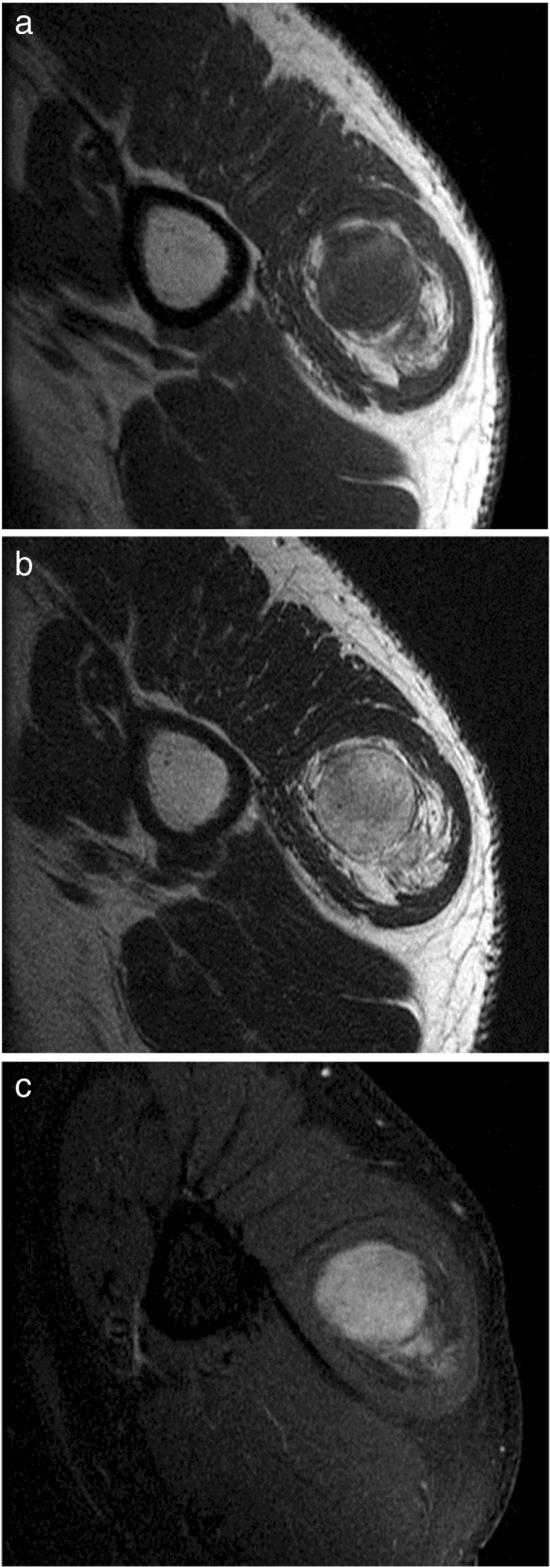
Signal intensity of the tumor on magnetic resonance imaging scans. (a) On this T1-weighted image, the tumor shows low signal intensity or isointensity to skeletal muscle at the center and high signal intensity at the periphery of the lesion. (b) On this T2-weighted image, the tumor shows high signal intensity. (c) On this gadolinium-enhanced image, the tumor shows high signal intensity.
